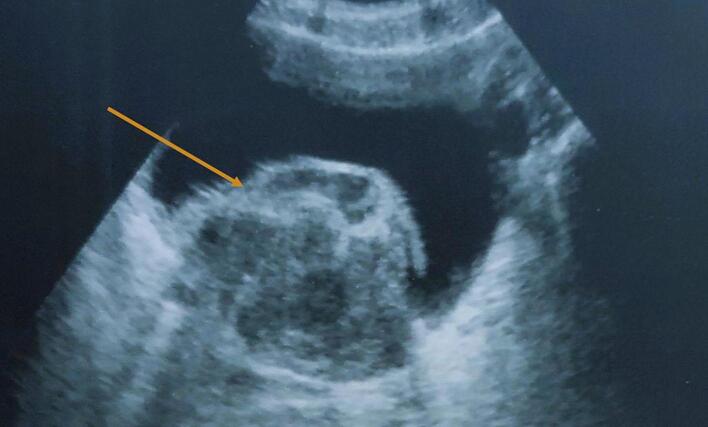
Image of a transverse ultrasound scan of the bladder revealing a circumferential, endoluminal and proliferative bladder wall thickening measuring 38.
